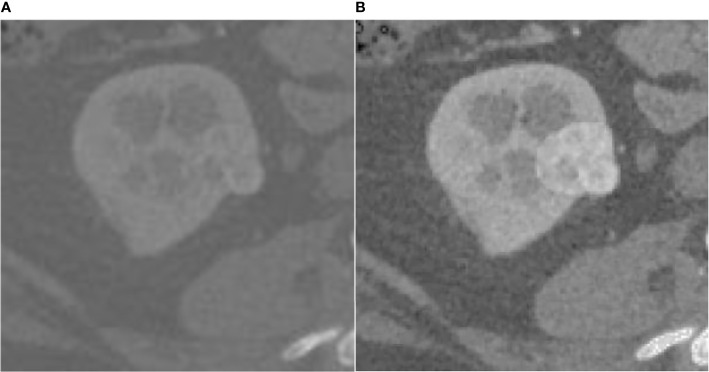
Result of fuzz set enhancement algorithm, (A) is the original CT image, (B) is the image enhanced by the fuzzy set.
